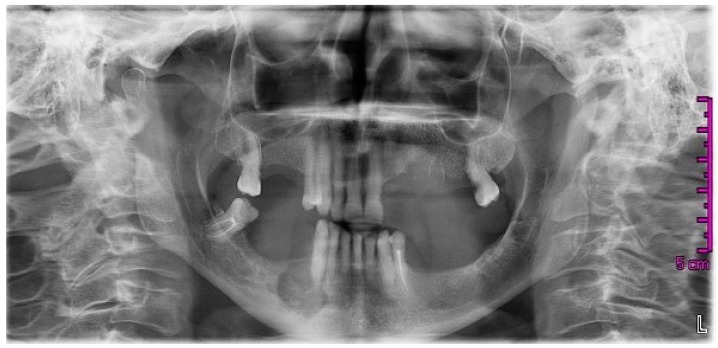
Orthopantomography dated October 2014, after extractions of teeth 24, 25, and 44. A wide radiolucent area involving the bone distal to 43 was present together with less-defined alterations in the upper left quadrant.
